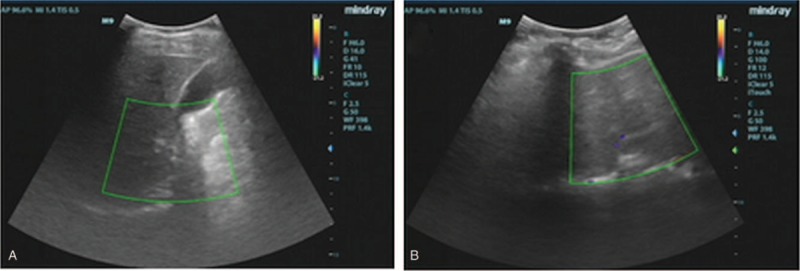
The type-B ultrasound results from the patients. There were no obvious abnormalities in the kidneys of Patient 1 (A) or Patient 2 (B).
